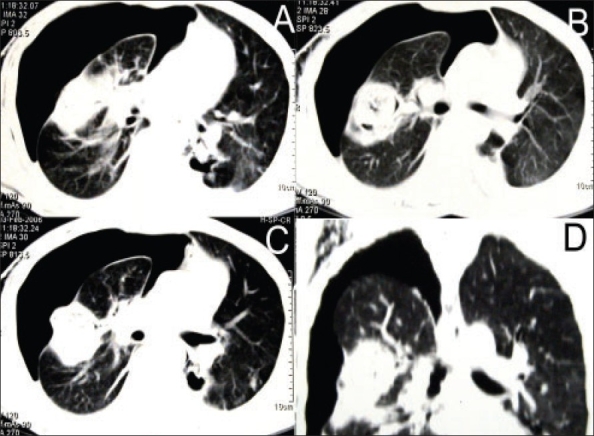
Computed tomogram of the thorax showing the various views of the mycetoma with rupture into the pleural cavity leading to pneumothorax.
